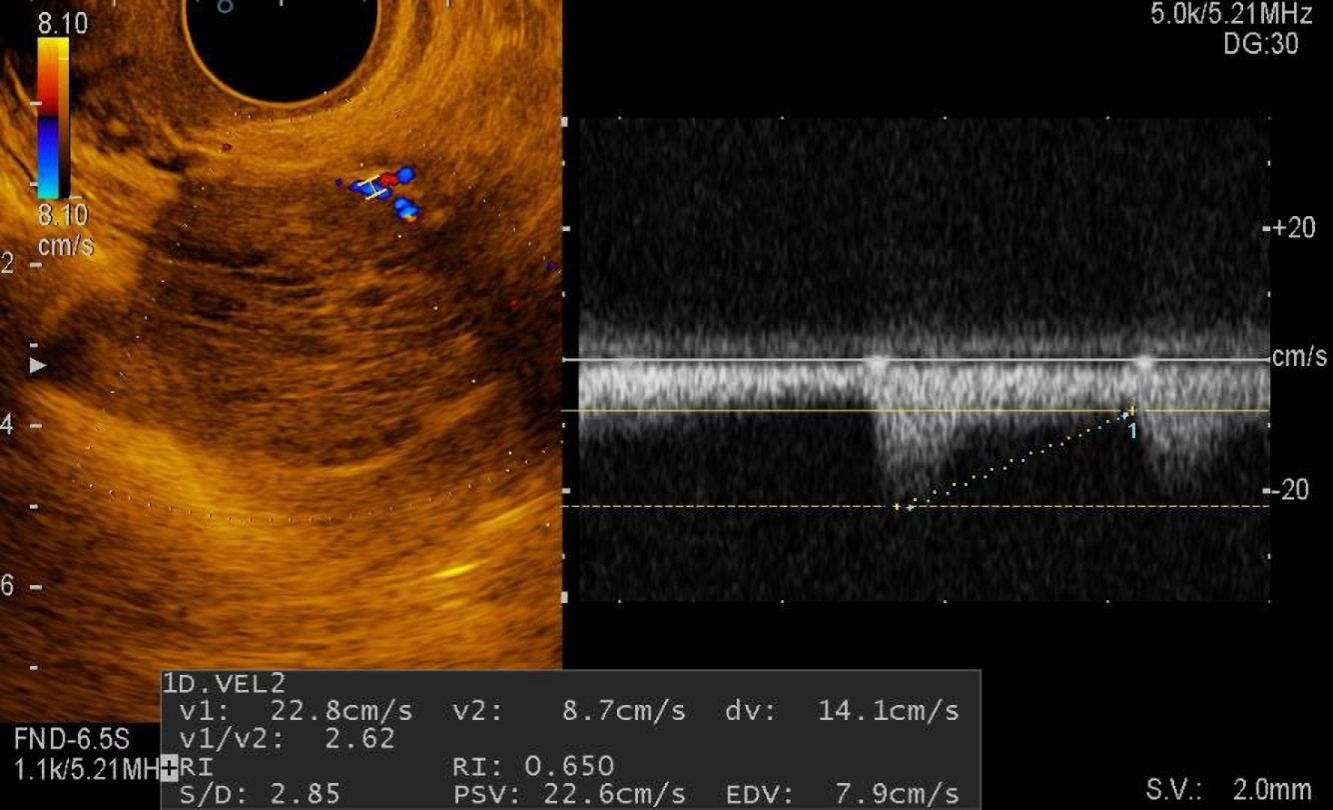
Sonogram of a corpus luteum cyst with Doppler ultrasonography.
